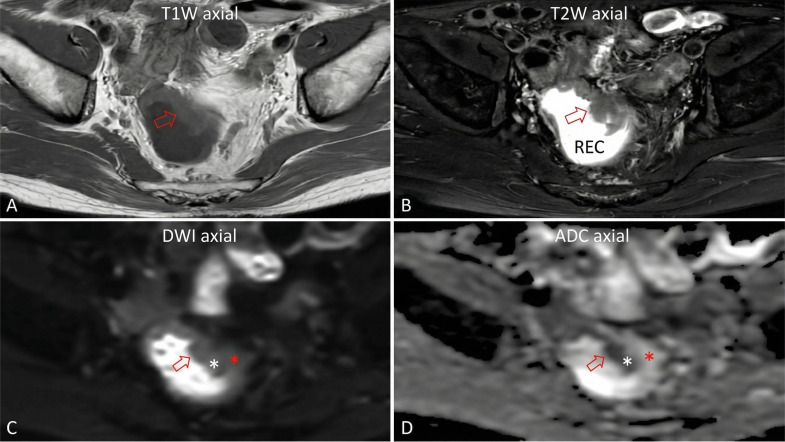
Axial pelvic MRI with different pulse sequences: (A, B) Axial T1W and T2W images show the tumor appearing isointense on both T1W and T2W images (red open arrow). (C, D) Diffusion MRI reveals two distinct tumor regions: (1) the surface demonstrates mild diffusion restriction, appearing slightly hyperintense on the DWI (gray) and hypointense on the ADC map (black) (white asterisk); and (2) the base shows no diffusion restriction, appearing hypointense on the DWI image (gray) and hyperintense on the ADC map (bright) (red asterisk). REC, rectum; T1W, T1-weighted; T2W, T2-weighted; DWI, diffusion-weighted imaging; ADC, apparent diffusion coefficient.
